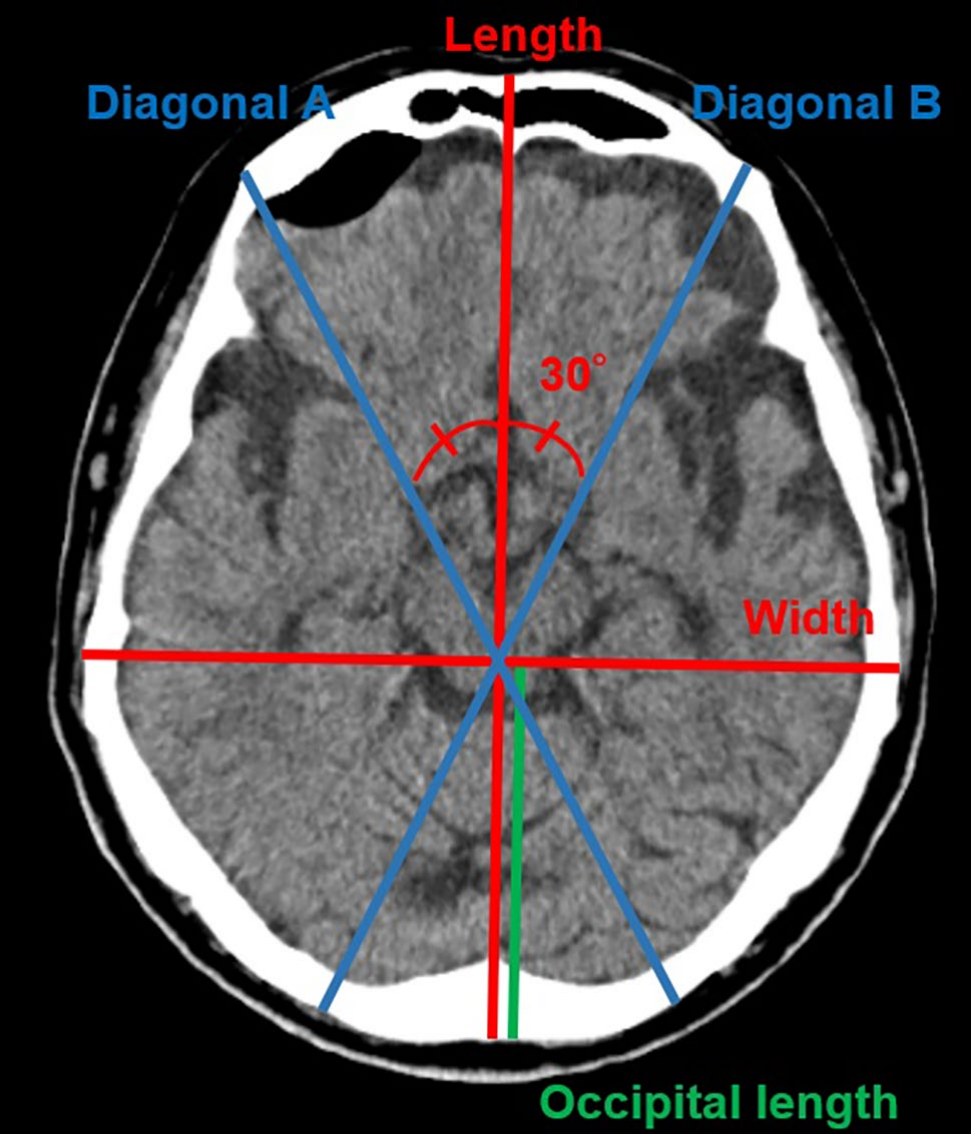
Cranial computed tomography image showing representative lines used to calculate the cephalic index, cranial vault asymmetry index, and occipital index.
